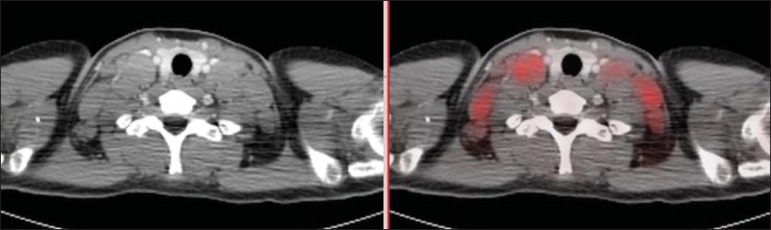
Pre-chemotherapy PET/CT image showing hypermetabolic lymph node enlargement
